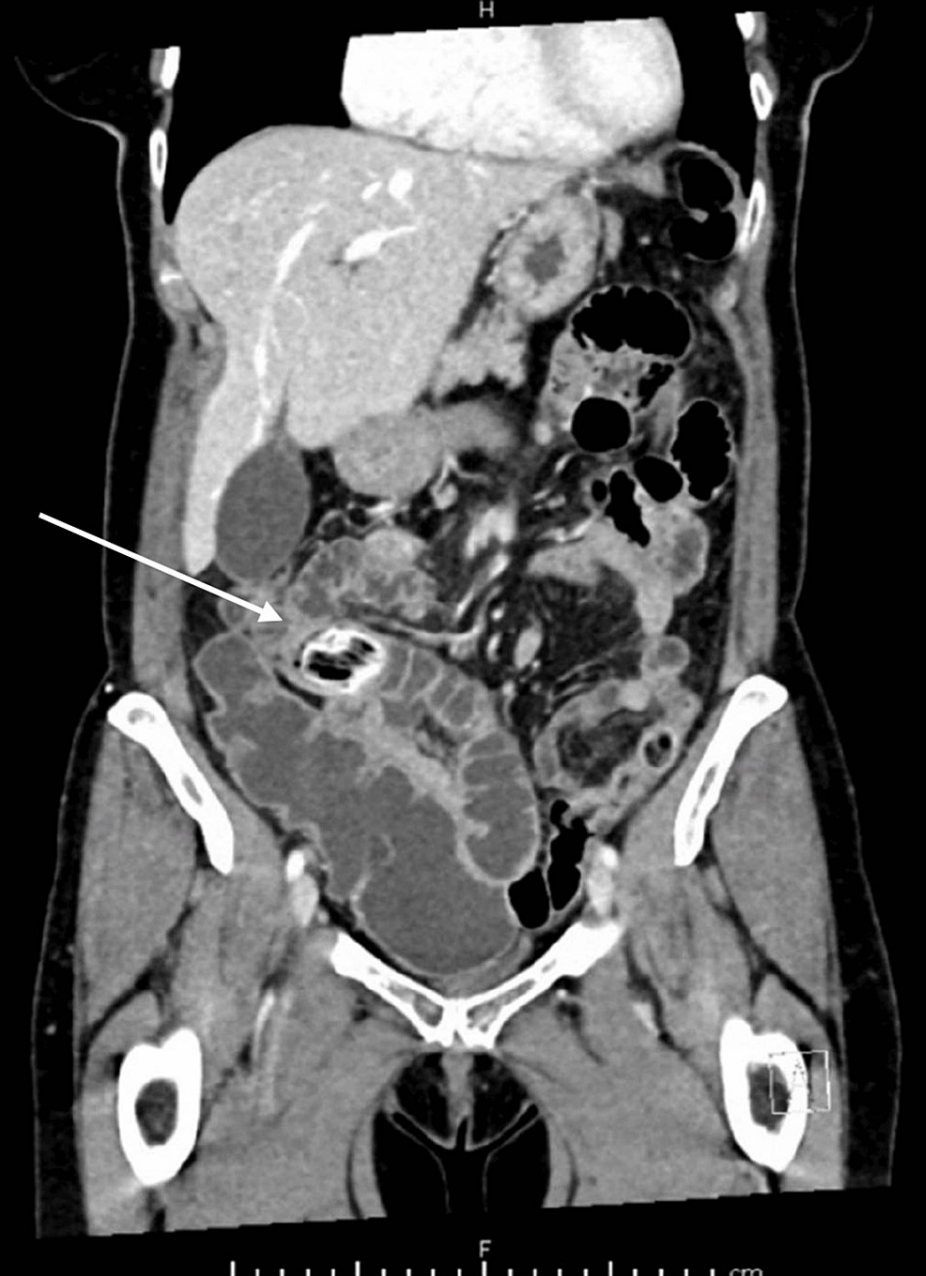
Coronal view of CT AP CT AP: Computed tomography scan of the abdomen and pelvis The image shows the presence of a jejunal diverticulum and a radio opacity, likely to represent an enterolith (white arrow).
